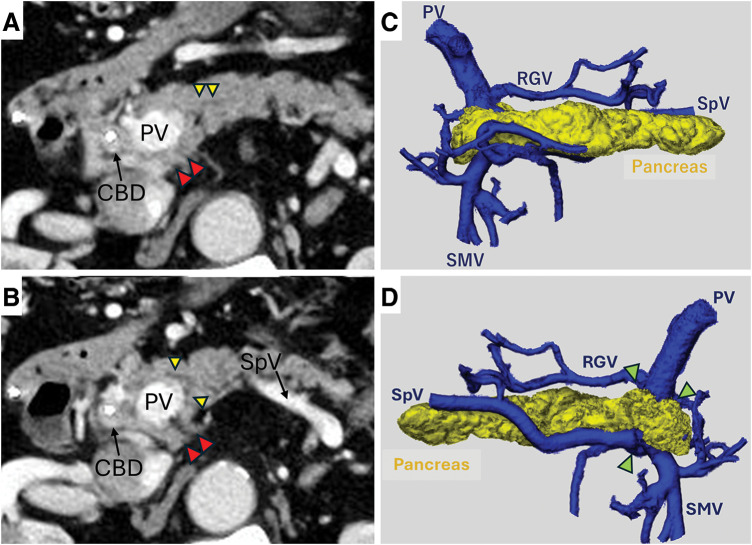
Contrast-enhanced CT and 3D CT findings of CP. (A) Thickened CBD wall with a biliary stent. Aberrant pancreatic parenchyma extending posterior to the PV corresponds to the characteristic findings of CP (red triangles). (B) No dilation of the MPD (yellow triangles) is detected, and its course within the pancreatic head remains indeterminate, making Joseph's classification inconclusive. (C) Anterior view of 3D CT shows pancreatic parenchyma encircling the PV. (D) Posterior view reveals the fusion plane cranial to the SpV (green triangles), consistent with Kawasaki’s type A classification. CBD, common bile duct; CP, circumportal pancreas; MPD, main pancreatic duct; PV, portal vein; RGV, right gastric vein; SMV, superior mesenteric vein; SpV, splenic vein
